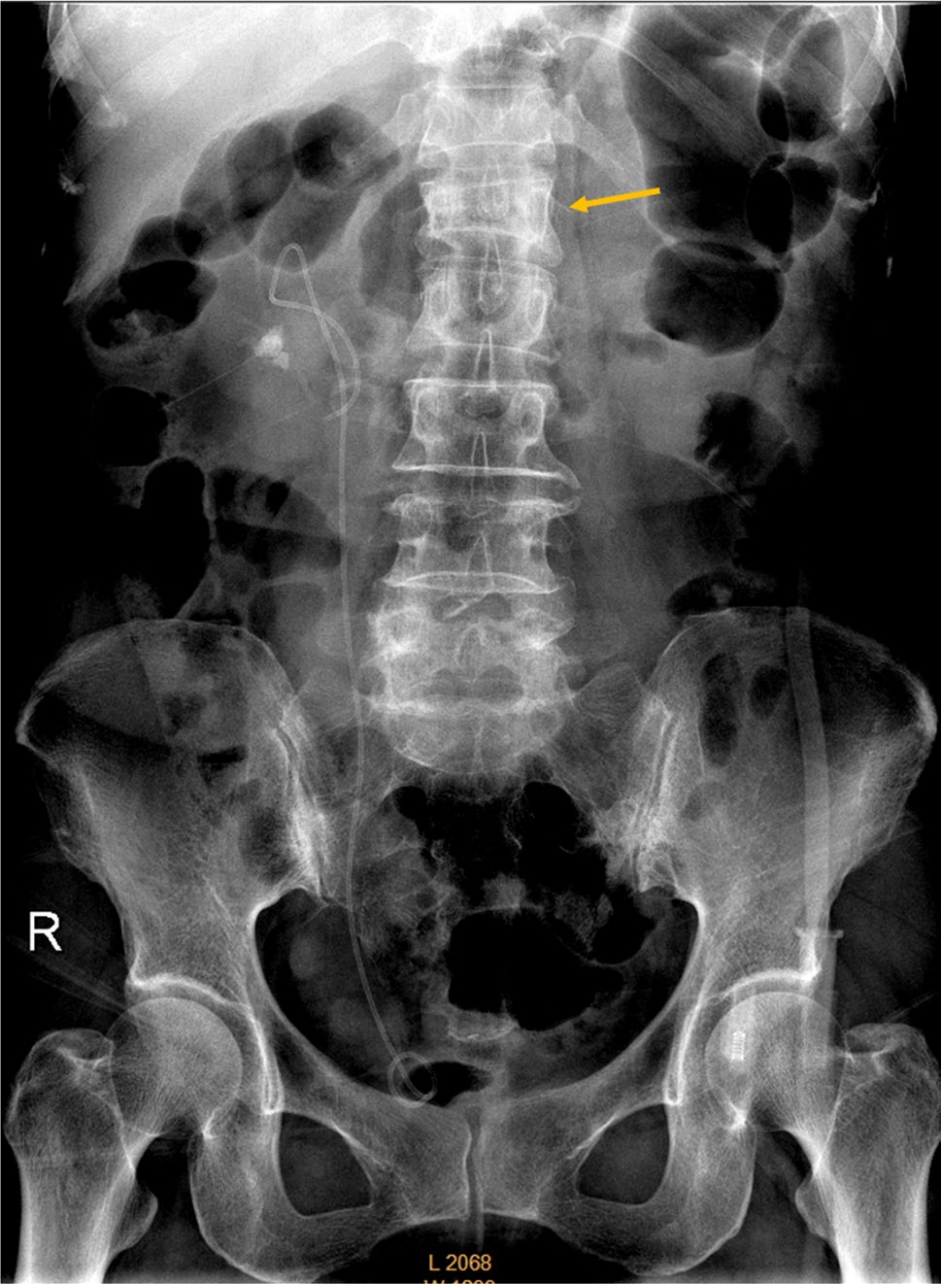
Abdominal radiograph of a 70-year-old female patient. A renal stone and double J catheter are noted on the right side. The arrow indicates an apparent L1 osteoporotic-like vertebral fracture. *Reproduced with permission from Du *et al*. J Orthop Translat. 2021;28:169–178*
